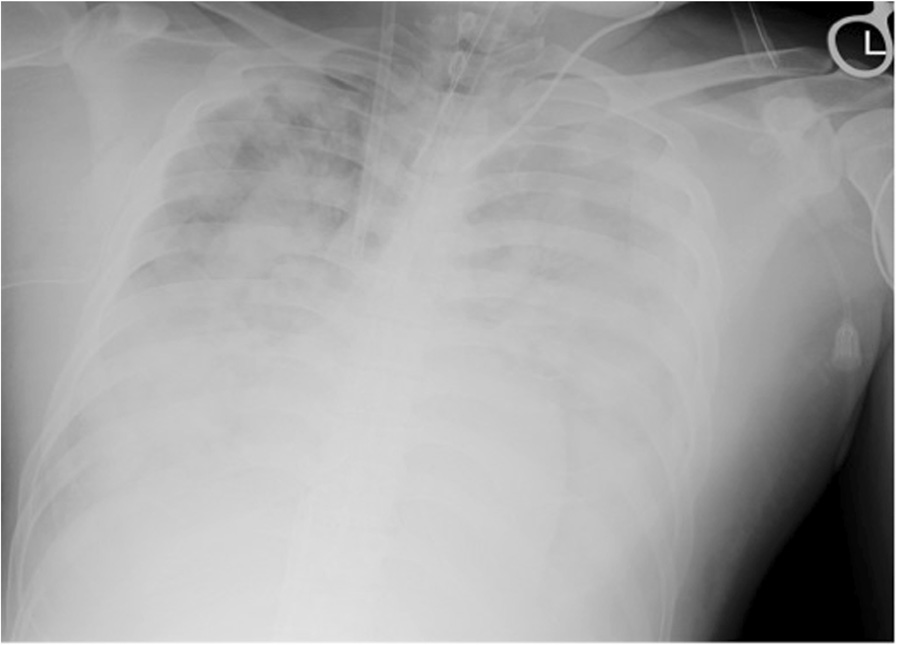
Chest radiograph at the time of initiating extracorporeal membrane oxygenation therapy.
